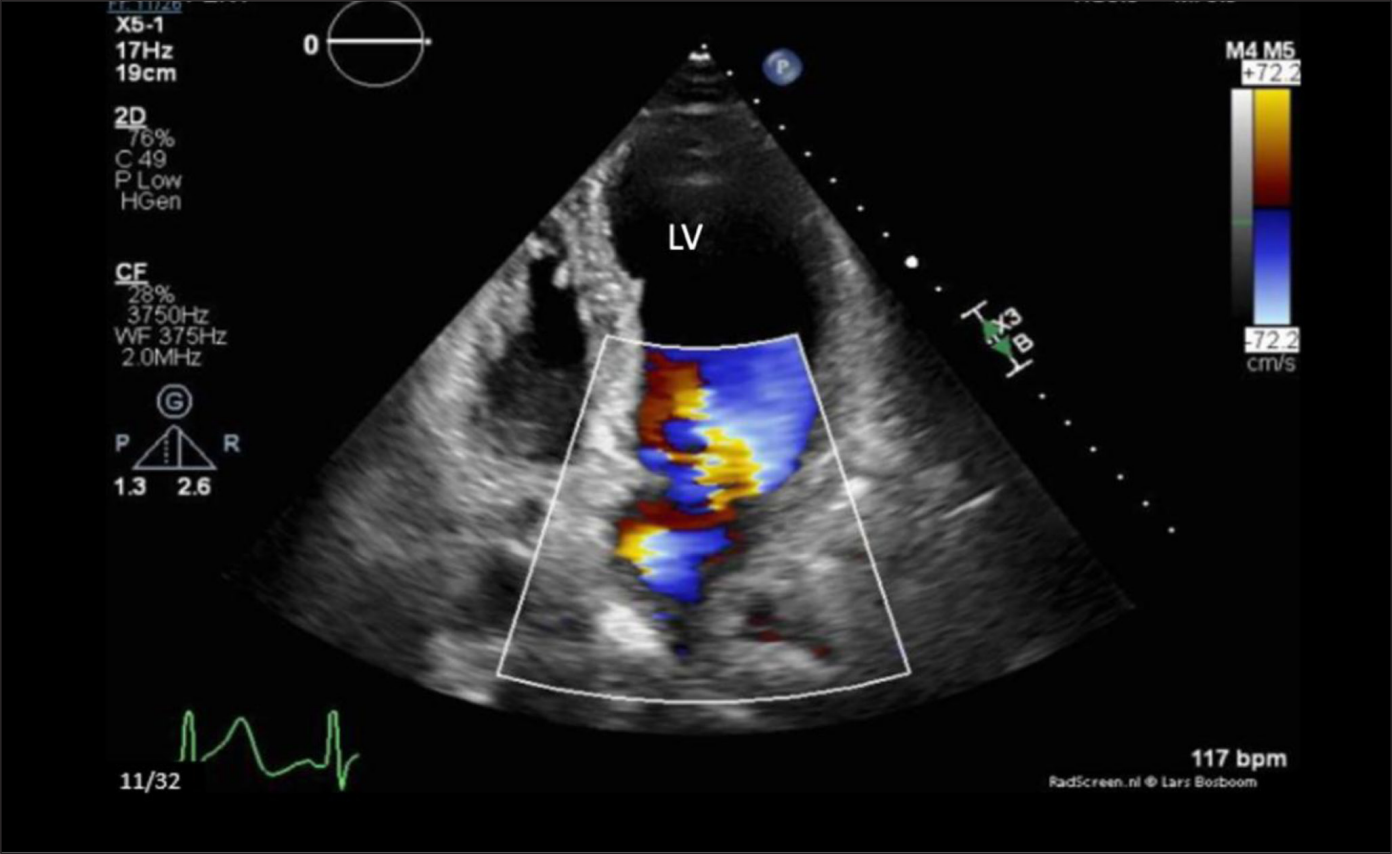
Transthoracic echocardiogram with apical four-chamber view in systole, showing severe jet of mitral valve regurgitations.
